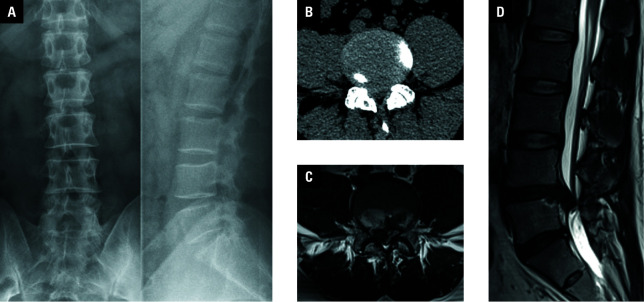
Preoperative images of a 38 -year-old man presenting with huge lumbar disc herniation (LDH) in the L4–L5 segment; A – X -ray image showing lumbar spine degeneration with narrowing of the intervertebral space at the L4–L5 level; B – computed tomography scan showing LDH in the L4–L5 segment; C, D – magnetic resonance imaging scans visualizing huge LDH occupying more than 50% of the spinal canal and causing severe compression of the dural sac and nerve roots in the L4–L5 segment
